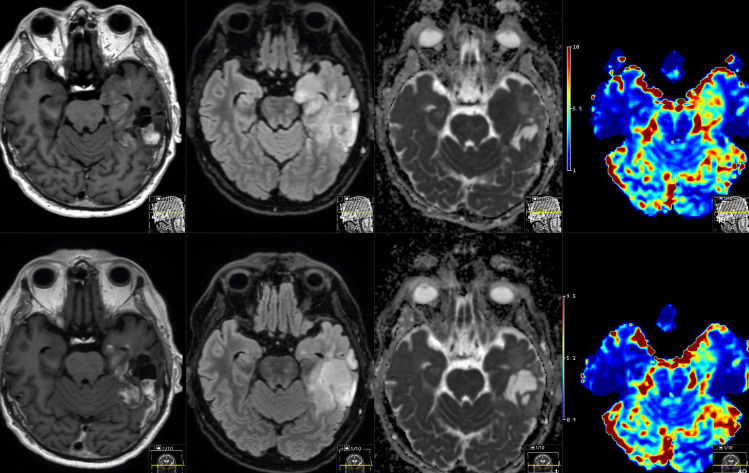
Patient with a glioblastoma investigated during treatment surveillance and Bevacizumab treatment. Lower row, before Bevacizumab treatment, and upper row after Bevacizumab treatment. After treatment, the contrast enhancement is slightly less pronounced at T1 post contrast images (left column), while the T2-FLAIR signal changes still continues to progress (second column from the left), the ADC-signal is not facilitated as would be expected in areas with less cell density, and the perfusion images shows that there still is hyperperfused progressing tumor areas
